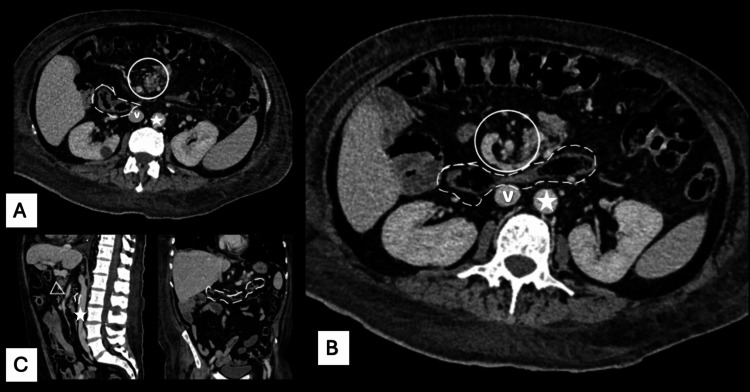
Transverse colon (A, B) Contrast-enhanced CT in the venous phase demonstrating the transverse colon (dashed line) crossing from right to left posterior to the mesenteric vessels (circle in A and B) and anterior to the inferior vena cava (V in A and B) and aorta (star in A, B, and C). (C) Sagittal and coronal reconstruction demonstrating the normal origin and course of the superior mesenteric artery (triangle) (dashed line: transverse colon; star: aorta).
